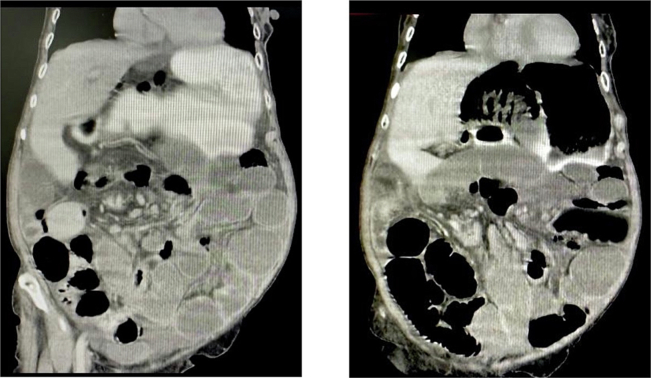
Abdominal CT: Trans-mesenteric internal hernia associated with internal obstruction and intestinal ischemia.
